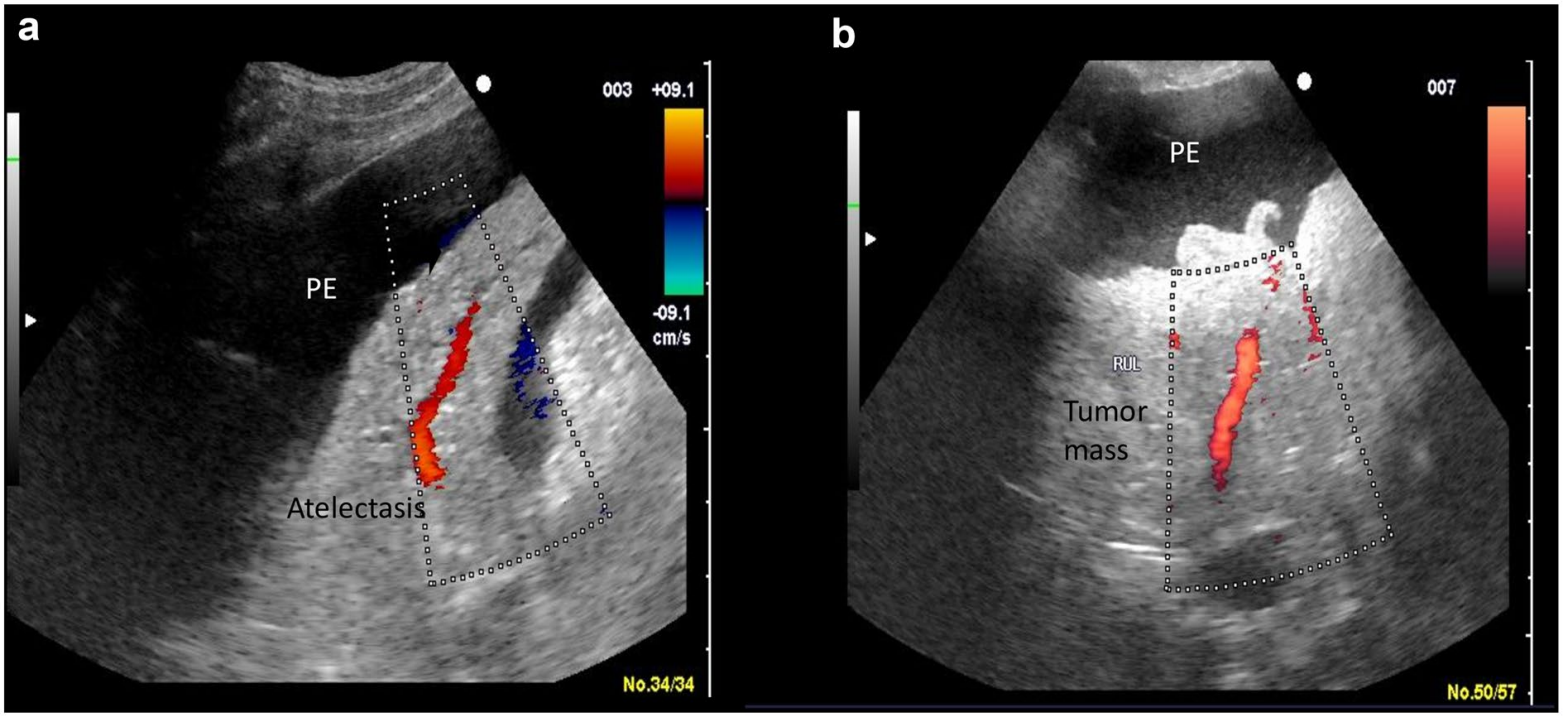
a Compressive atelectasis associated with large pleural effusion (PE). Colour doppler was used to depict the vessel inside the consolidated lung. b Right upper lobe tumor. Notice the augmented vascularization, the irregular boundaries and the complete loss of aeration. *PE* pleural effusion
